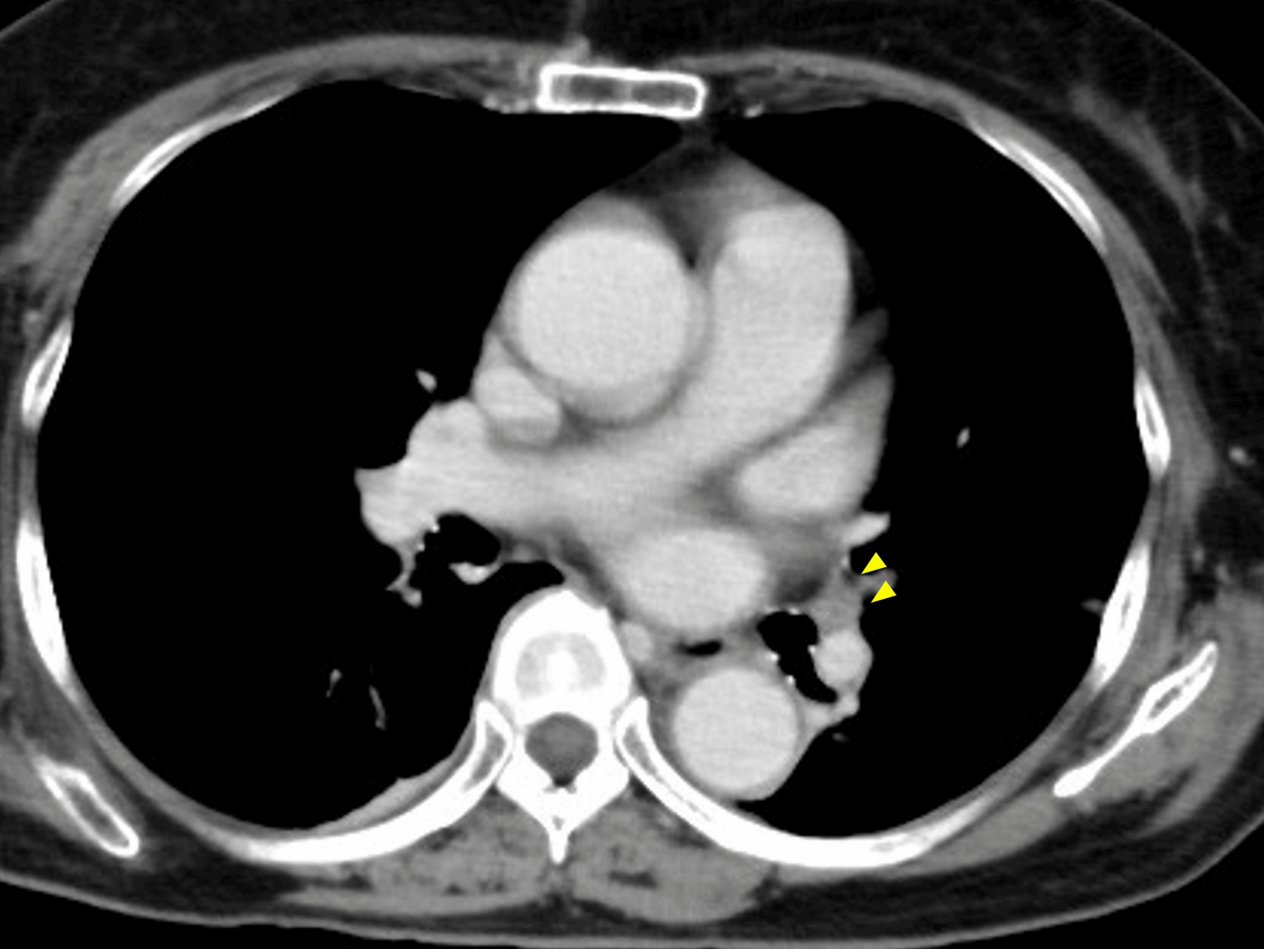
Computed tomography angiography findings in Case 1 Chest computed tomography angiography revealed blood flow interruption in the left pulmonary artery due to embolization (arrowhead).
